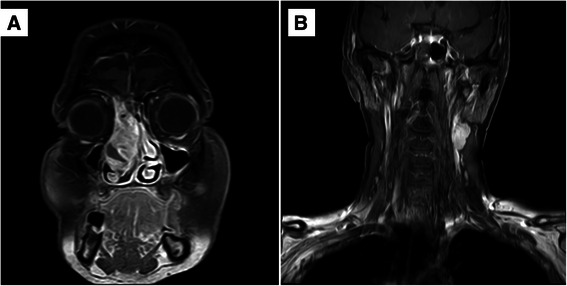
Magnetic Resonance Imaging of the Primary Tumor and Lymph Node Metastases. November 2008, coronal MRI with contrast of the head showing the primary tumor in the right main nasal meatus and ethmoid sinus (a). December 2009, coronal MRI with contrast of the head and neck demonstrating two spherical, enlarged cervical lymph nodes on the contralateral side (b)
